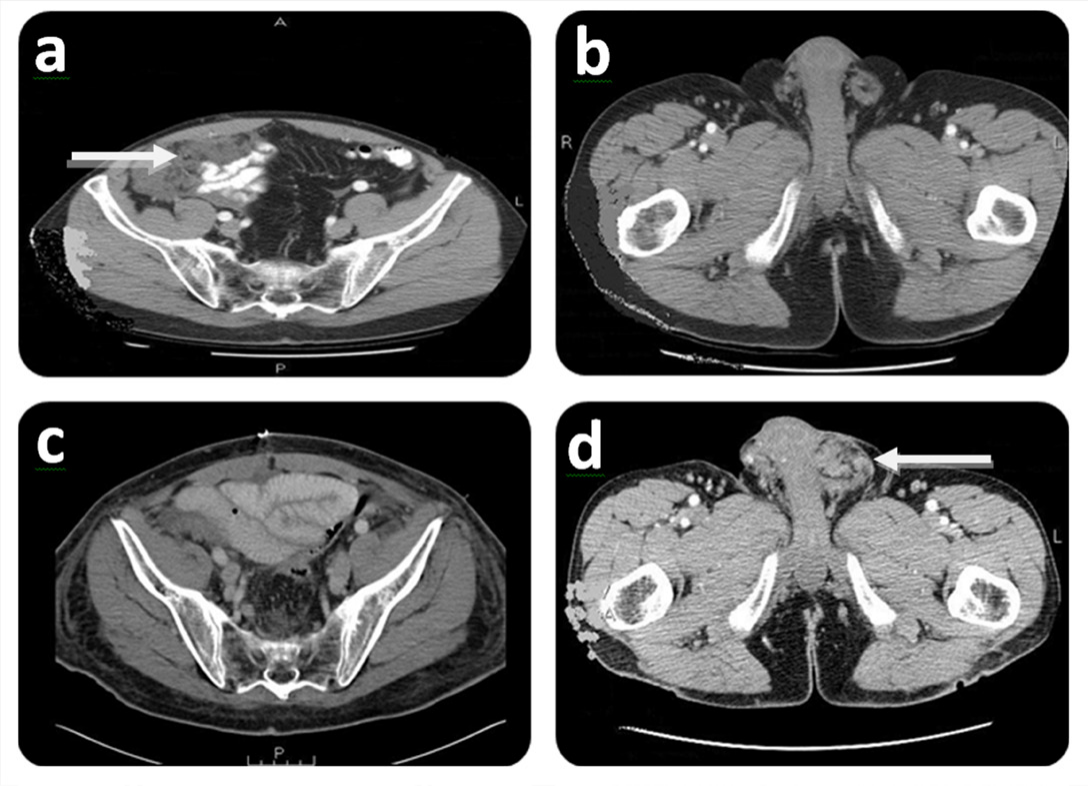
Initial and follow-up CT scan findings of Case # 2. Preoperative: A) CT scan shows right lower abdominal mass (white arrow) at initial CRS/HIPEC. A: Anterior; P: Posterior. B) CT scan shows no groin involvement at initial CRS/HIPEC. R: Right side; L: Left side. Postoperative (7 months after CRS/HIPEC): C) CT scan shows no evidence of disease at time of scrotal recurrence. P: Posterior. D) CT scan showing left scrotal mass (white arrow). L: Left side.
